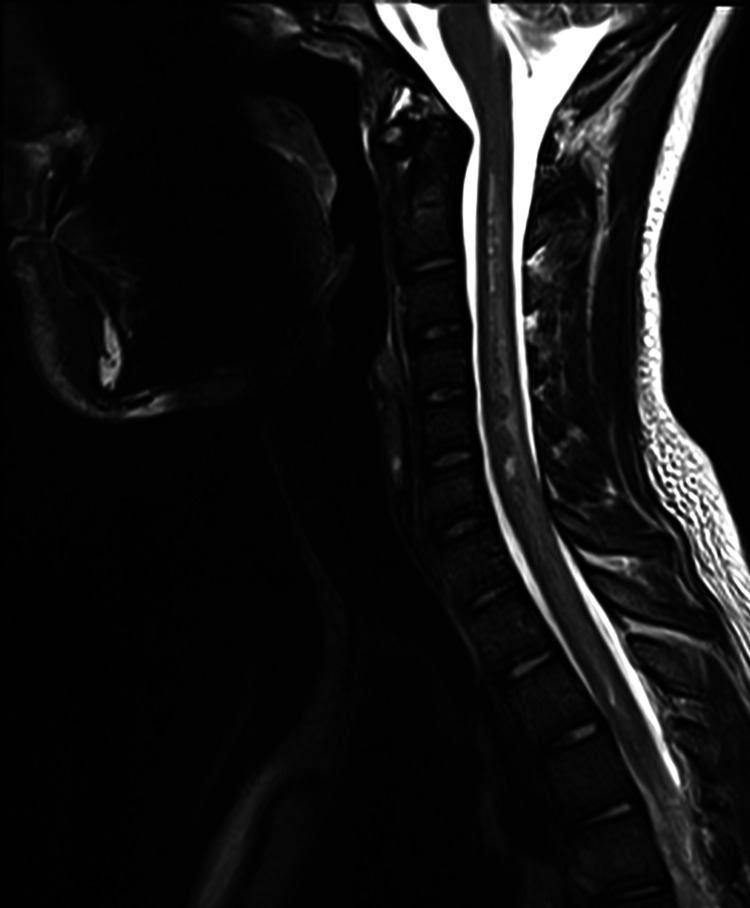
Sagittal T2-weighted MRI of the cervical spine demonstrating swelling and edema of the spinal cord.
